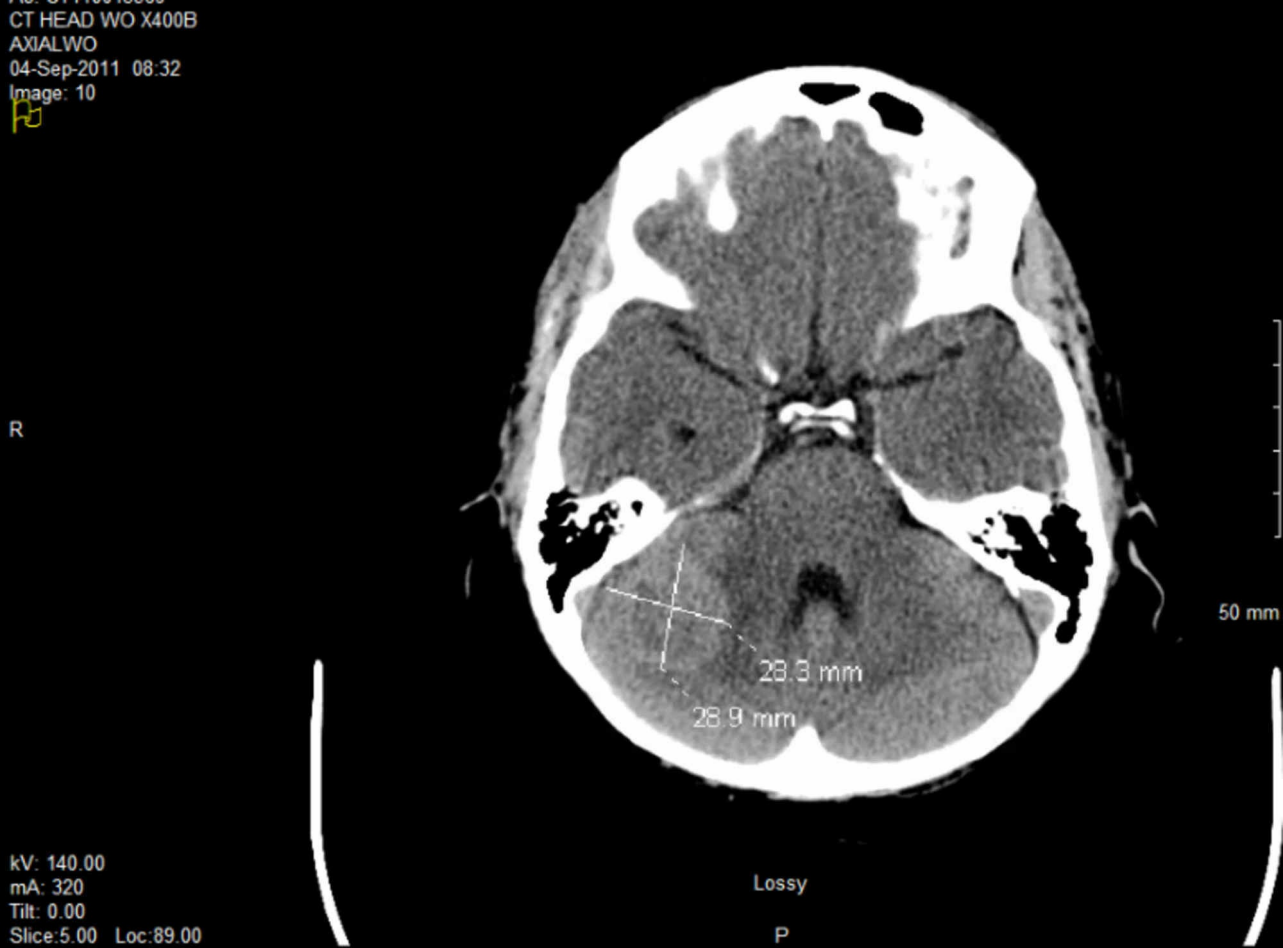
Brain CT showing a large mass in the right cerebellum before the first WBI in 2011 WBI, whole brain irradiation
